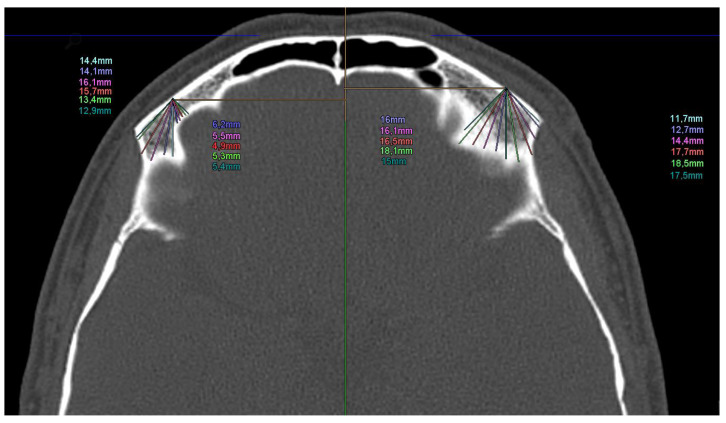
Axial section of a clinical CT scan at the level of the supraorbital insertion area with the measured angles and corresponding potential monocortical drilling depth.
